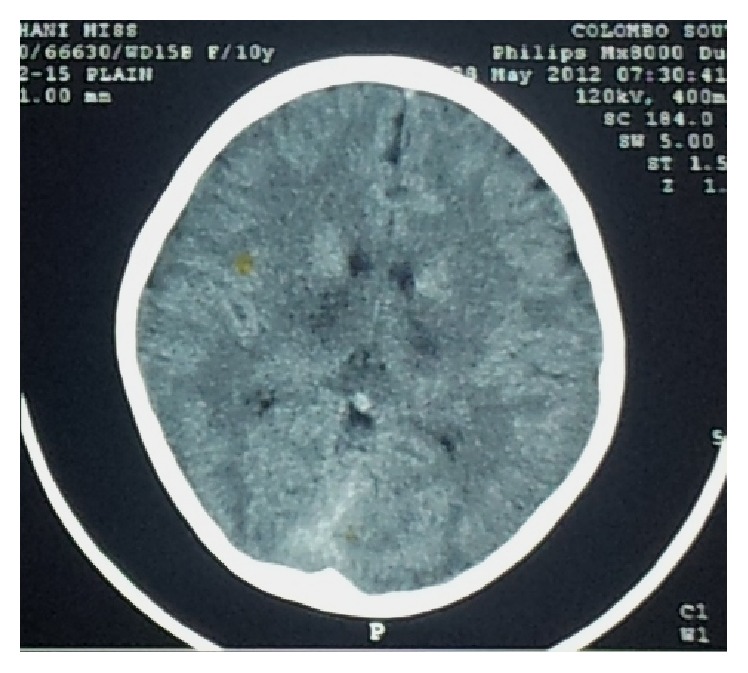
Axial brain CT image (with contrast) showing hypodense areas in the region of thalamus and basal ganglia.
